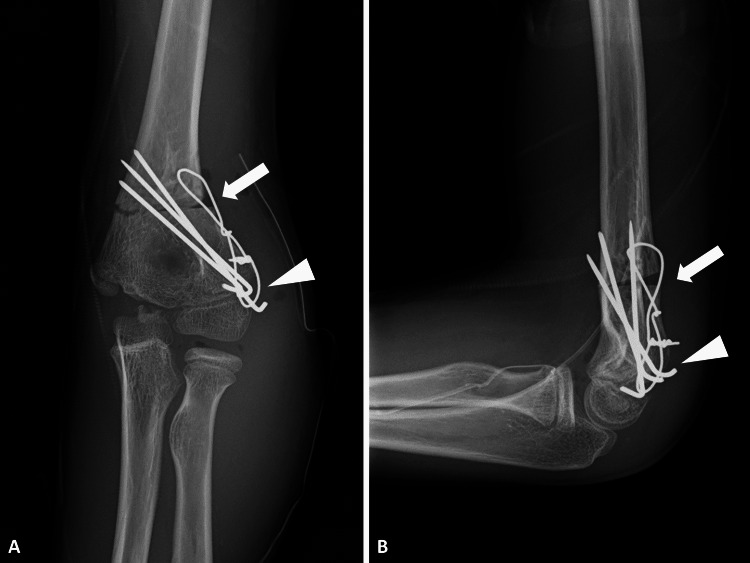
Plain radiography after operation of open reduction and corrective osteotomy. (A) Anteroposterior and (B) lateral views of the left elbow after operation. (Arrows: osteotomy site; Arrowheads: fracture site).
